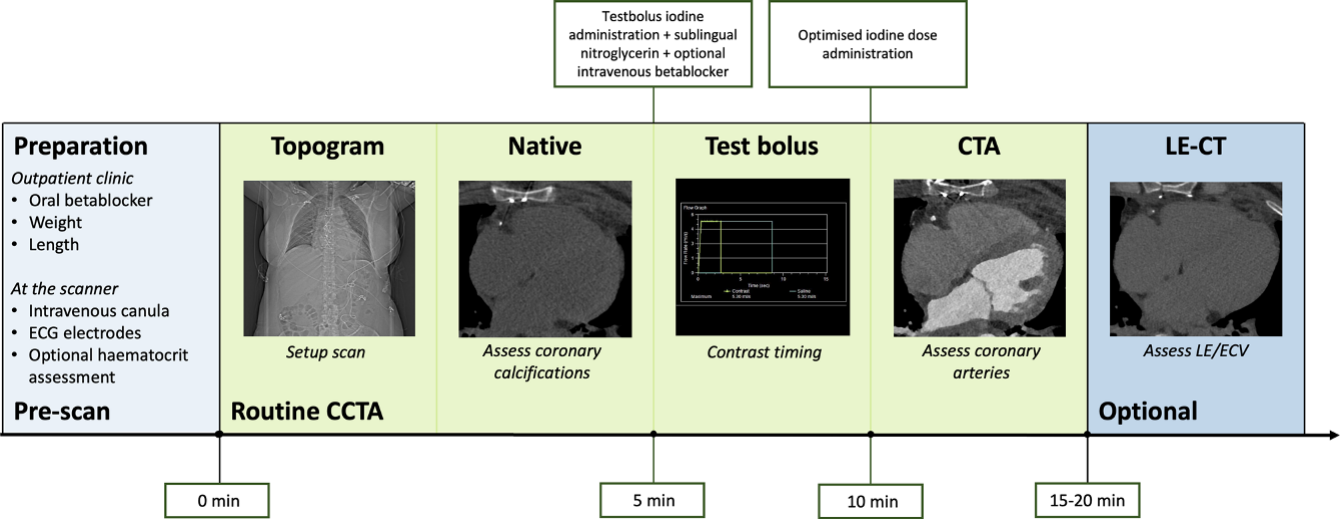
Photon-counting detector CT imaging protocol as a one-stop-shop for both coronary artery assessment and late enhancement imaging. The duration of a regular coronary CT angiography (CCTA) protocol is approximately 10 min, an additional late enhancement (LE) CT acquisition extends the total duration to approximately 15–20 min. Acquisition times may differ between centres. A haematocrit assessment is only necessary for the optional extracellular volume (ECV) assessment acquired 5–10 min after contrast administration. A native scan is optional as it can be reconstructed as a virtual non-iodine image
